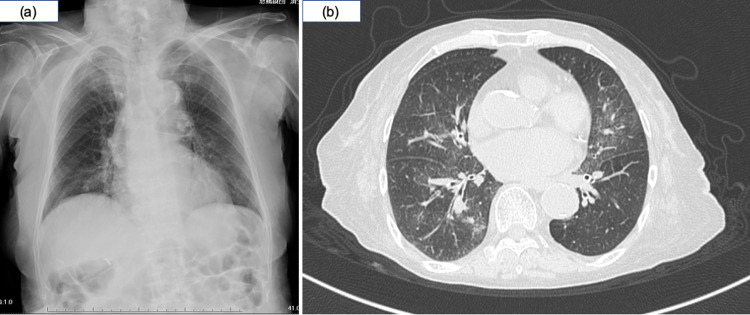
Ground glass opacity in the bilateral lower lobe on chest X-ray (a) and CT (b)
